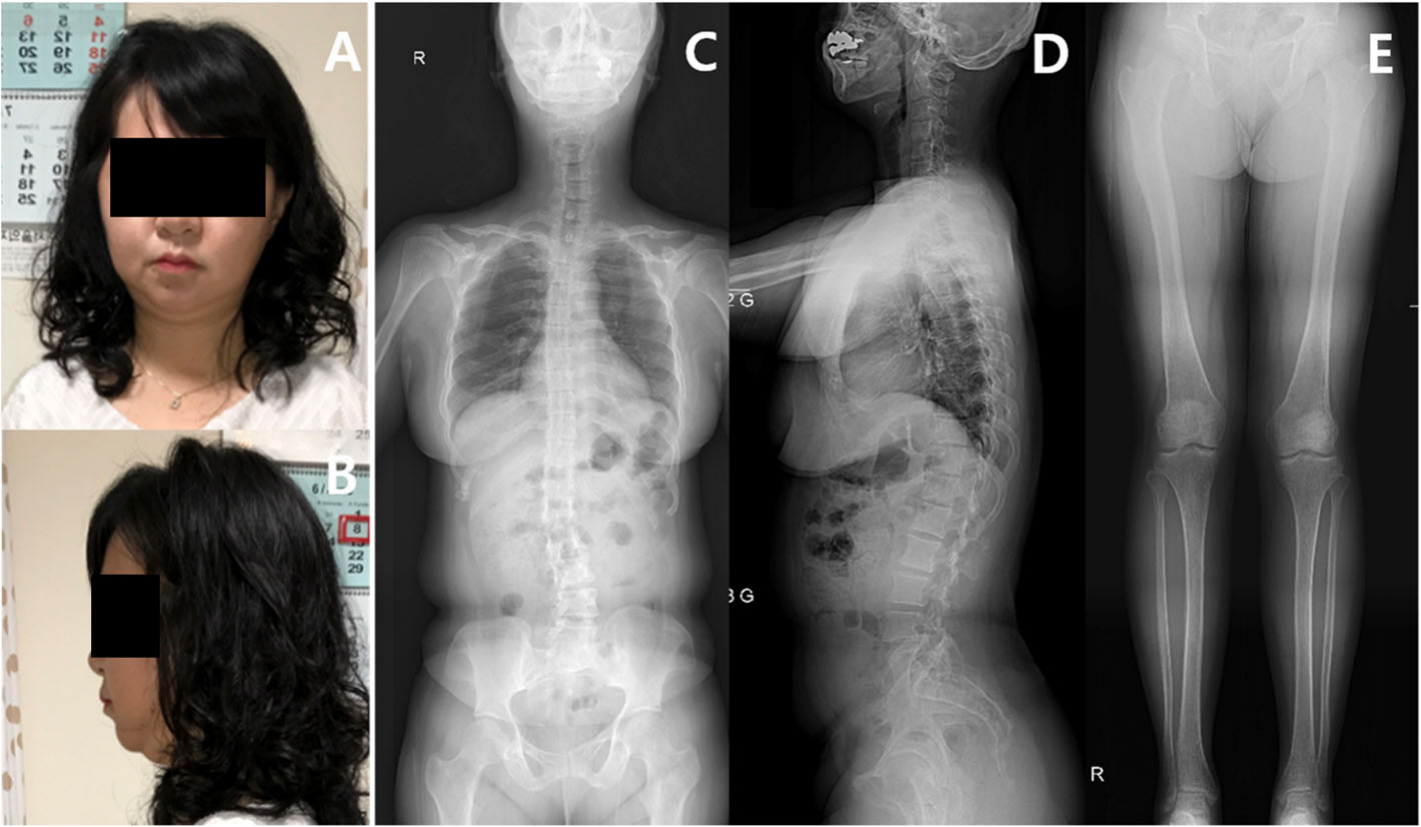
Photographs and plain radiographs of the patient’s mother. Overall, the patient’s mother had relatively mild deformities, when compared with the patient. a, b Like the patient, she had full cheeks and micrognathia,which were mild. c, d, e She also had mild lumbar scoliosis, kyphosis, and minimally bowed legs bilaterally
